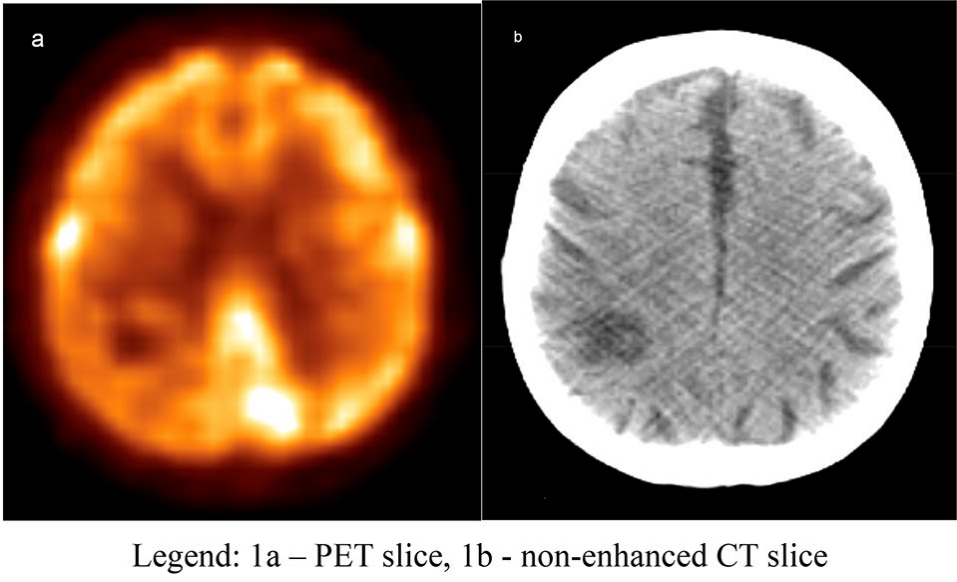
54 y.o. patient scanned with the [18F]FDG PET/CT (CUP syndrome). Abnormal finding within the right parietal lobe showing a lesion with decreased [18F]FDG uptake surrounded by the brain tissue oedema (*source*: original figure; software—Philips Fusion Viewer). (a) PET slice, (b) non-enhanced CT slice.
